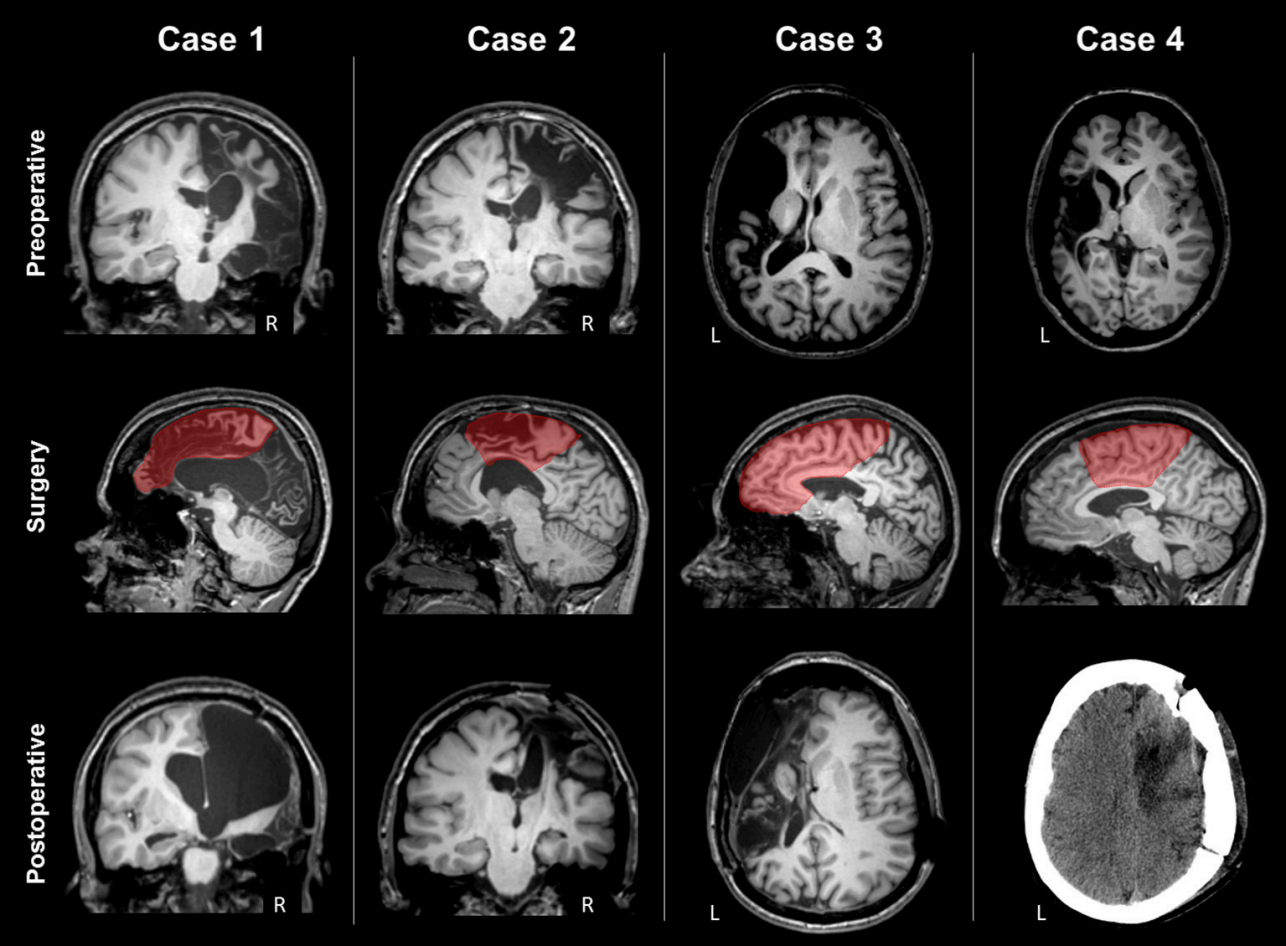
Preoperative MRI, type of surgery and postoperative scan of the four patients. Case 1 presented severe atrophy of the right hemisphere; rolandic convolutions were partially spared, and CST partially recognizable. The type of surgery was right frontal lobectomy. Postoperative MRI showed that the right CST was not recognizable anymore. Case 2 presented a right frontal malacic area partially sparing the motor cortex and the right CST. The type of surgery was a right fronto-central cortectomy with disconnection. Postoperative MRI showed removal of the upper part of motor cortex and degeneration of the right CST. Case 3 presented a large left frontal malacic area, sparing the upper part of the motor cortex, but involving the posterior limb of the internal capsule, and the CST. The type of surgery was right frontal lobectomy. After surgery, frontal convolutions were not recognized anymore. Case 4 presented a large left malacic area sparing the upper part of the motor cortex, but involving the internal capsule and the CST. The type of surgery was a right fronto-central cortectomy with partial disconnection, as observed in the postoperative CT scan.
